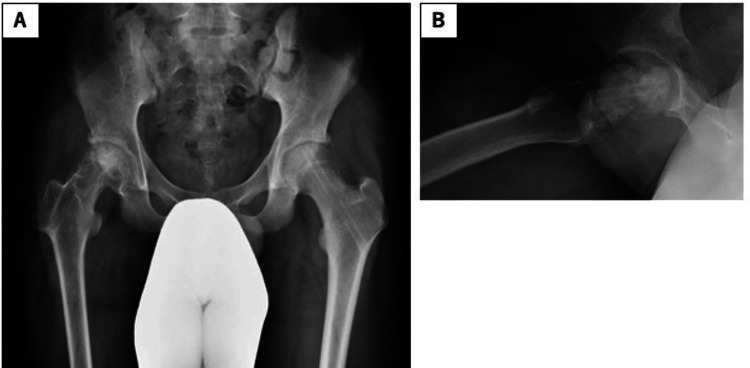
Plain radiographs at final follow-up (4.5 years postoperatively) revealing epiphyseal closure, without severe femoral head deformity. (A) Anteroposterior view; (B) lateral view.
